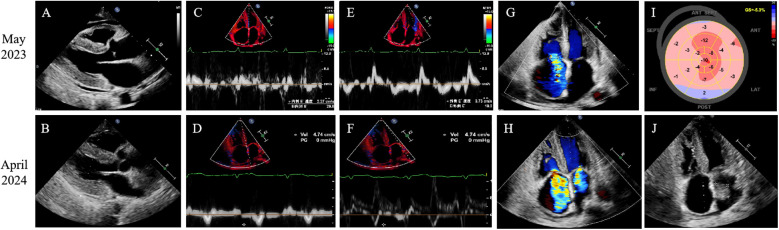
Transthoracic echocardiogram images. (A,B) Parasternal long-axis views revealed LV thickness of 13 mm and right ventricular thickness of 7 mm. (C–F) Transmitral Doppler imaging revealed decreased Sep e′ and Lat e′. (G,H) The colour Doppler flow imaging showed tricuspid regurgitation. (I) Left ventricular global longitudinal strain analysis indicated strain of 5.3%, with prominent apical sparing. (J) The apical four-chamber view showed a left atrial thrombus (29*25 mm).
